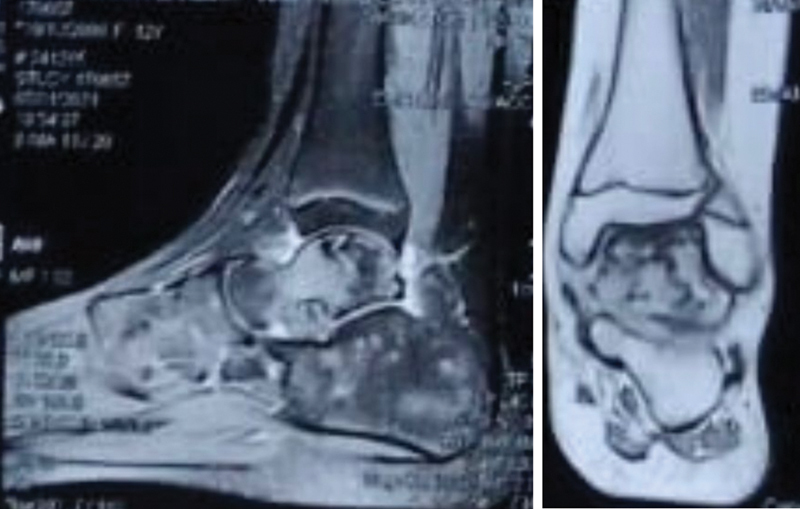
Magnetic resonance imaging of the ankle. Source: Service collection.
